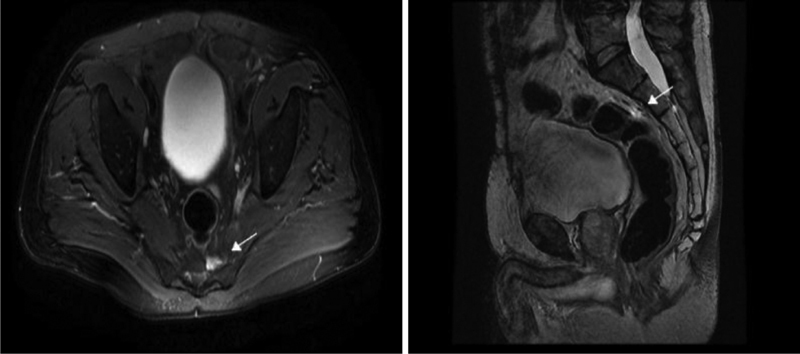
MRI (after 3 months): High contrast material uptake approximately 1 cm diameter in the anatomical position of the excised lesion (
